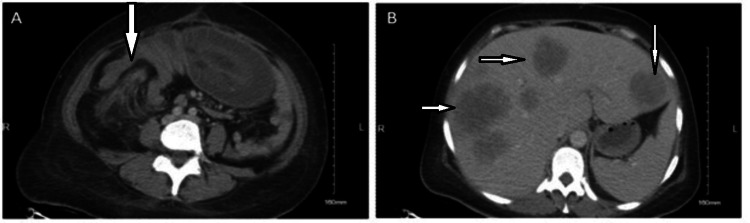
CT scan images of the patient A. CT scan image showing the ileocolic intussusception (arrow) in the 48-year-old patient with Large Cell Neuroendocrine Carcinoma (LCNEC). B. The patient had multiple lesions in the liver (arrows) at presentation suggestive of hepatic metastasis.
